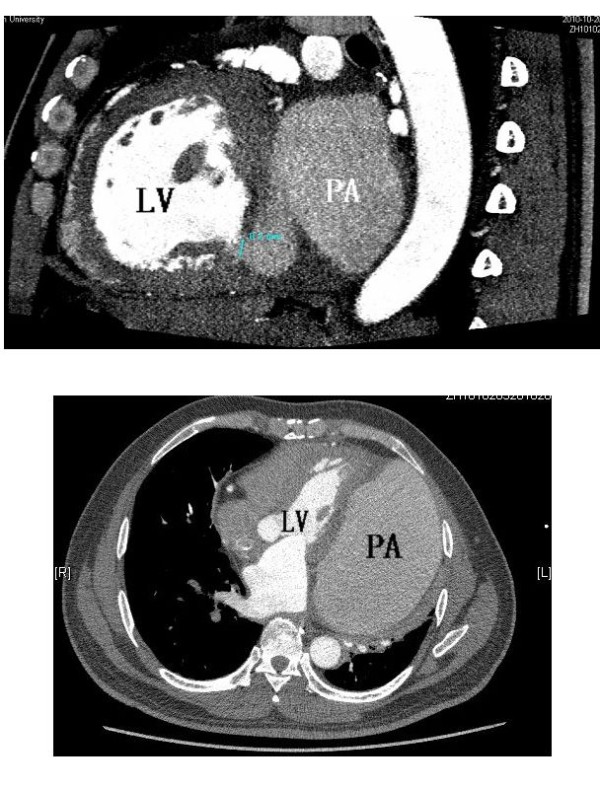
Preoperative cardiac computed tomography. PA, pseudoaneurysm.
